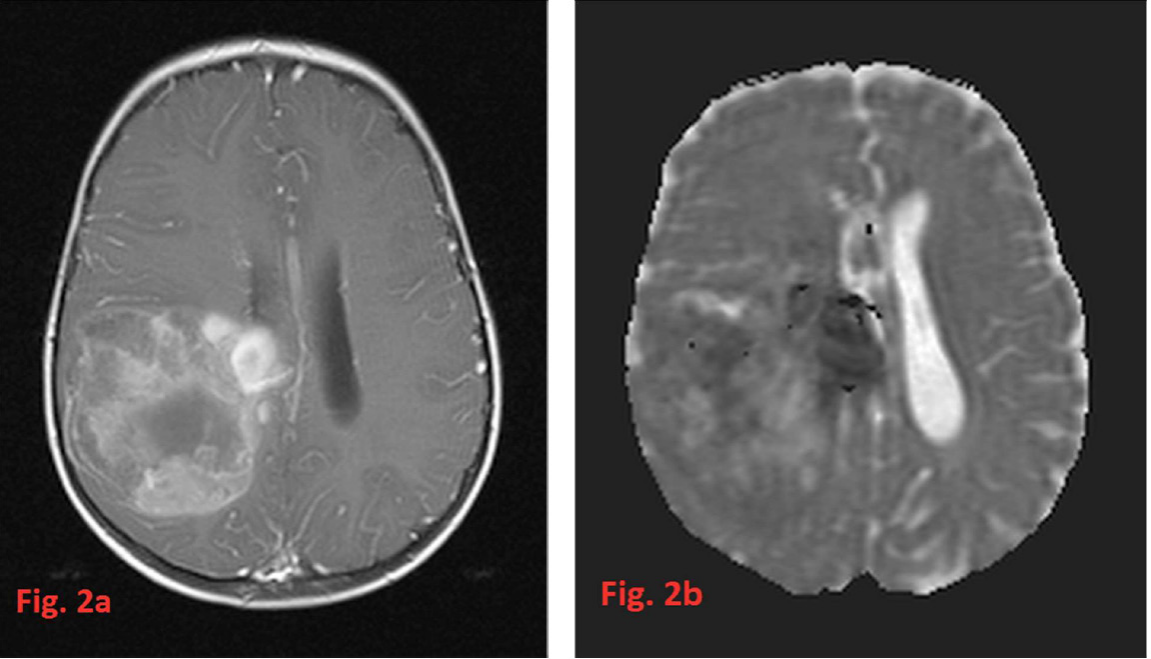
In a right frontoparietal large glioblastoma; enhancement on a T1-weighted MRI after contrast (a) is very inhomogeneous. The corresponding ADC-map (b) also shows a variability of restricted diffusion with the most restricted areas above the roof of the right lateral ventricle. On T1-weighted images before contrast (not shown) also met-hemoglobin as residue of subacute bleeding is present.
